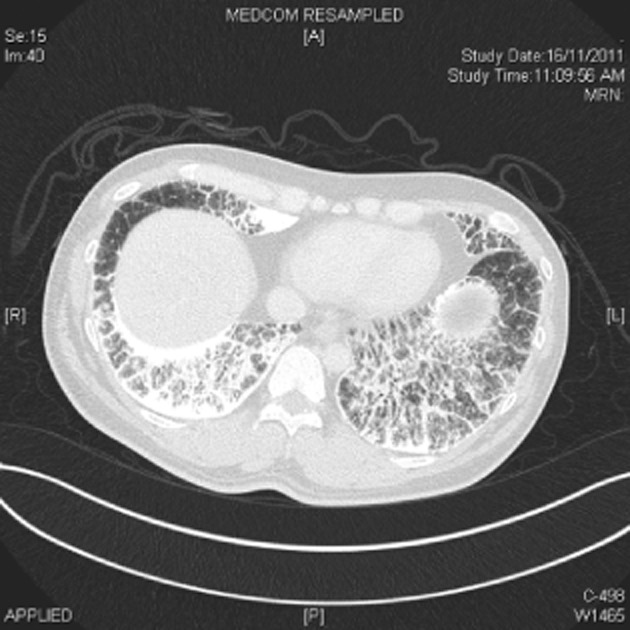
High-resolution computed tomography of thorax in lung windows showing septal thickening associated with pulmonary alveolar microlithiasis.
